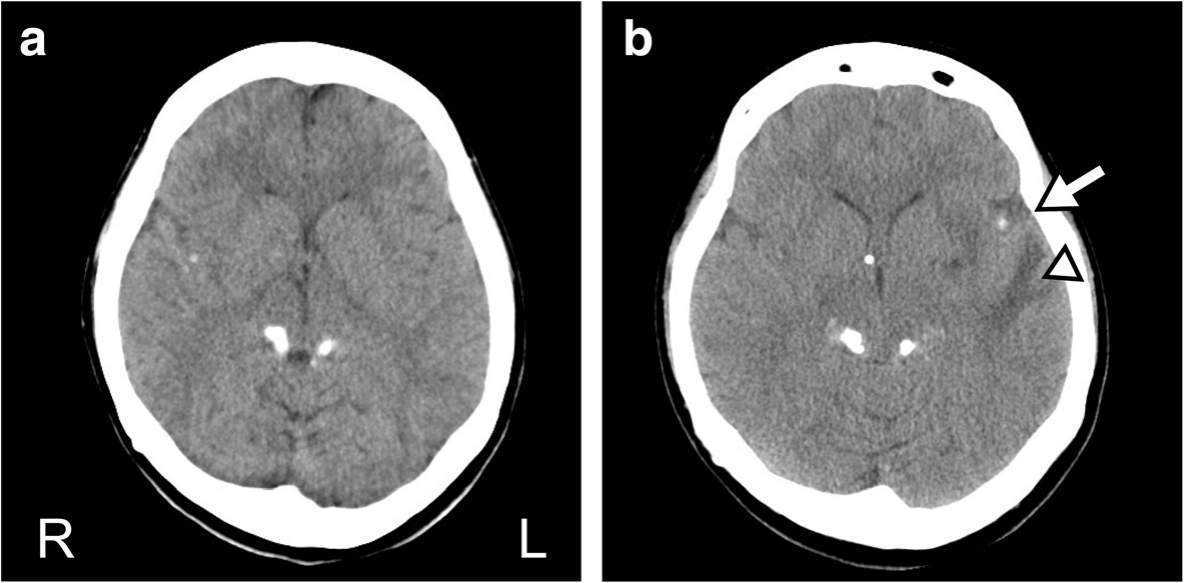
A head CT before and after the onset of PR. a, An axial head CT performed just after the completion of anti-TB therapy 10 years ago. Tuberculomas are visible in the bilateral ambient cistern. b, An axial head CT performed on admission. New emerging tuberculomas are visible in the left sylvian fissure (arrow), which are accompanied by a low-density area in the left temporal lobe (arrowhead)
